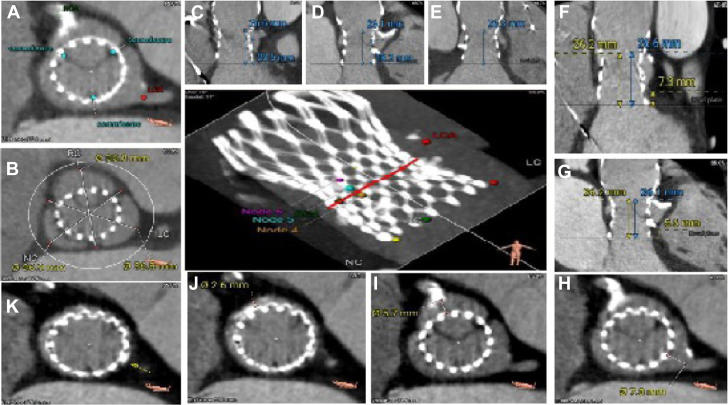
Computed Tomography Transcatheter Aortic Valve Replacement Measurements for Valve-in-Valve Procedure Planning (A) CoreValve commissures in relation to the left and right coronary arteries. (B) Sinus of Valsalva diameters. (C) Left sinus height. (D) Right sinus height. (E) Noncoronary sinus height. (F) Left coronary sinus approximate distances, including CoreValve inflow to native annulus, CoreValve inflow to sinotubular junction (STJ), and CoreValve pinned leaflet height with STJ, are 0.4 mm above the top of the pinned leaflet. (G) Right coronary sinus approximate distances, including CoreValve inflow to native annulus, CoreValve inflow to STJ, and CoreValve pinned leaflet height with STJ, are 0.1 mm above the top of the pinned leaflet. (H) Valve to left coronary artery distance. (I) Valve to right coronary artery distance. (J) Valve to STJ in right coronary artery direction. (K) Valve to STJ in left coronary artery direction.
